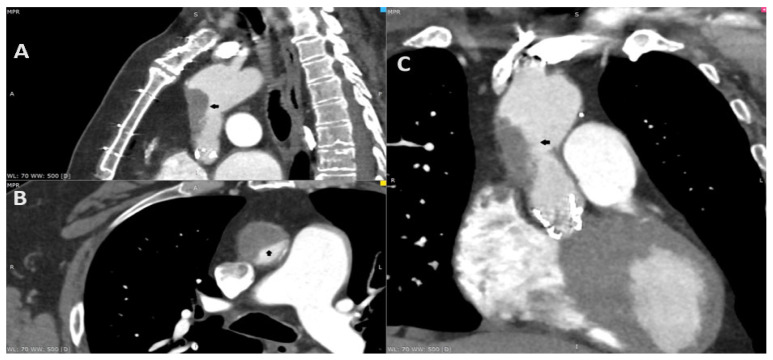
A 69-year-old male complaining of recurring chest pain, episodes of syncope and visual distortion was admitted to the hospital. His past medical history included the replacement of the ascending aorta with surgical aortic valve replacement (SAVR) in 2011 due to aortic aneurysm and aortic stenosis (Bentall procedure). He underwent transcatheter aortic valve implantation (TAVI) in 2021 due to the degeneration of aortic valve bioprosthesis, using a 26 mm balloon-expandable Sapien 3 Ultra (Edwards Lifesciences, Irvine, CA, USA). The treatment course after TAVI involved longitudinal single antiplatelet therapy (SAPT)—aspirin. In addition, he reported reduced exercise tolerance since the TAVI procedure. The coronarography in 2011 and angio-CT in 2021 excluded the significant coronary artery disease before operations. The angio-CT, including the aorta and head, at the current hospitalization found the thrombosis of the ascending aorta graft causing 80% stenosis (A–C; black arrow). Furthermore, due to the resolution of the neurological dysfunction, the patient was diagnosed with a transient ischemic attack (TIA). The transthoracic echocardiography revealed an increase in gradient through aortic valve bioprosthesis—a mean gradient of 24 mm Hg. The low-molecular-weight heparin (LMWH) in therapeutic dose was initiated. Despite the increase in cardiac enzymes, the chest pain and the diagnosis of non-ST elevation myocardial infarction (NSTEMI), the coronarography was not performed due to the risk involving the dislocation of the thrombi. After three days, the control CT displayed a significant reduction in the thrombus. The patient consulted with the Heart Team and was transferred to a higher reference clinical hospital with the availability of the cardiac surgery department.
